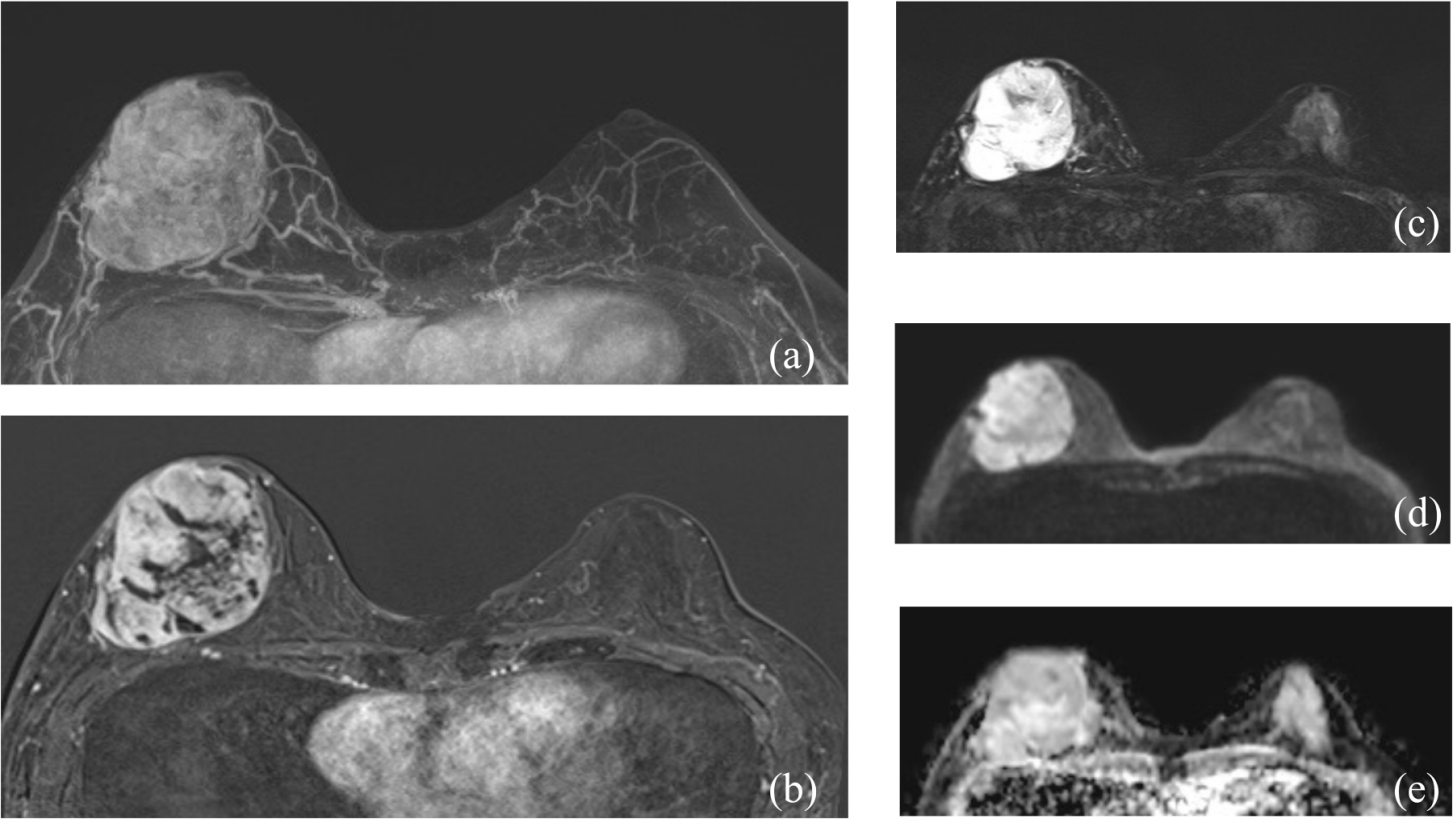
MRI findings in a 50-year-old female with metaplastic carcinoma with mesenchymal differentiation. (a-b) Axial Maximum Intensity Projection (MIP) and subtraction T1-weighted contrast-enhanced images display a large, round mass in the right breast, exhibiting heterogeneous enhancement and a circumscribed margin. (c) The axial T2-weighted image shows the mass with high signal intensity. (d-e) The diffusion-weighted imaging (DWI) and the corresponding Apparent Diffusion Coefficient (ADC) map reveal a high ADC value of 2.04 x 10^-3^ mm²/s.
